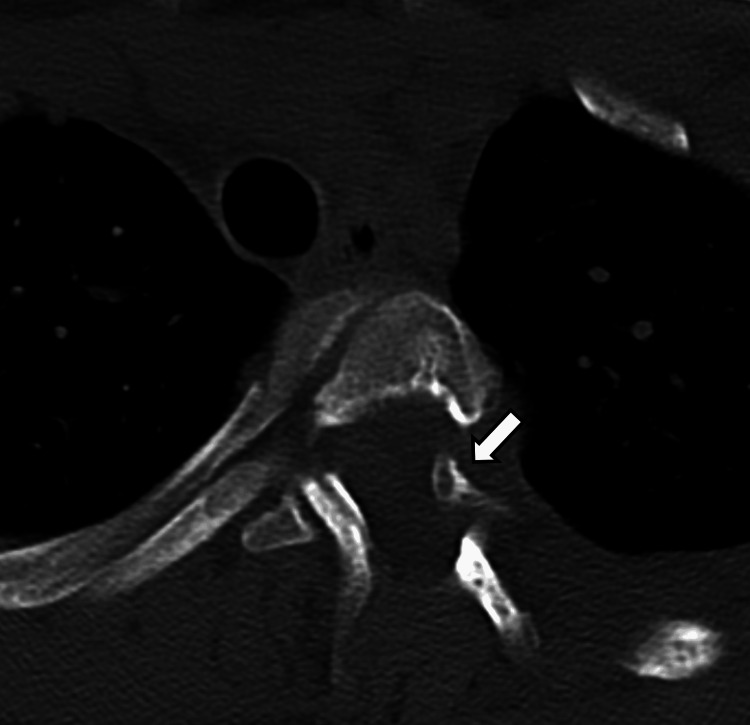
Axial CT images in bone kernel through the T3-T4 neural foramen demonstrate protrusion of the pointed head of the rib through the foramen into the spinal canal (arrow).
